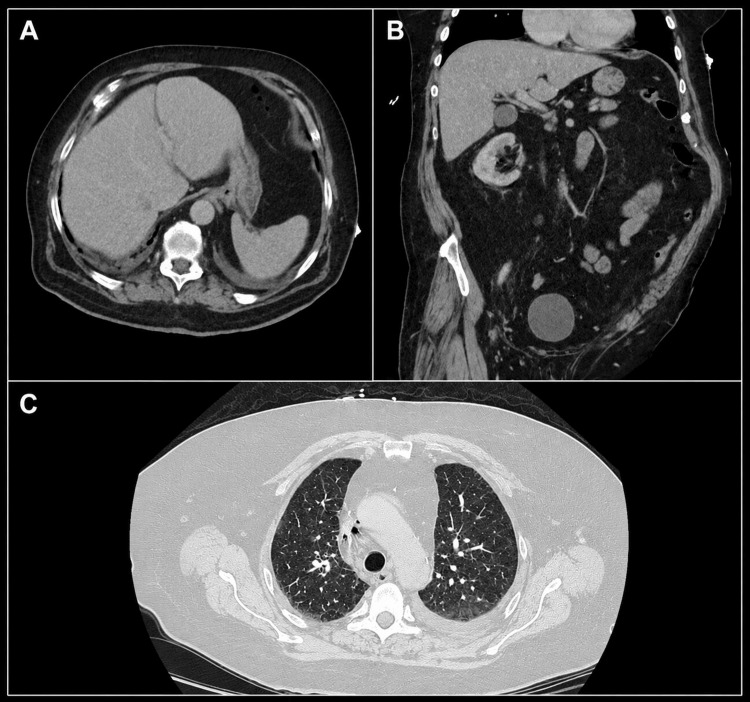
CT scan of the chest, abdomen, and pelvis (A) Axial CT image of the abdomen demonstrating no evidence of hepatic or intra-abdominal metastatic lesions. (B) Coronal CT image of the abdomen and pelvis demonstrating absence of distant abdominal or pelvic metastases. (C) CT image of the chest demonstrating no pulmonary metastases or suspicious large pulmonary nodules. CT: computed tomography
